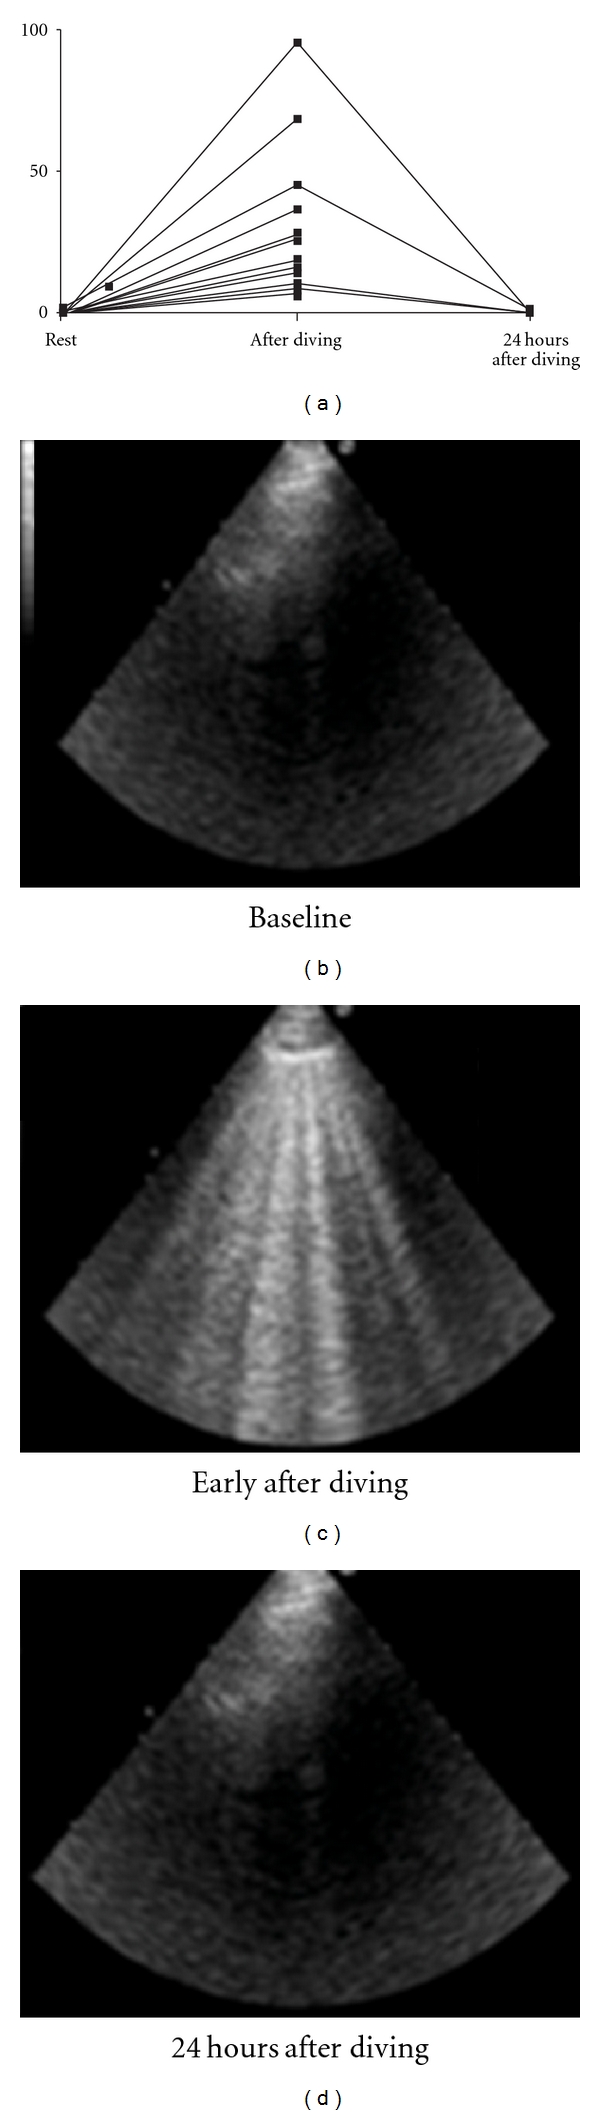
Chest ultrasonography revealed an increased number of ULCs within 10 minutes after immersion in 45% of 14 top-level breath-hold deep divers, which resolved completely after 24 hours (Frassi et al. [71]).
